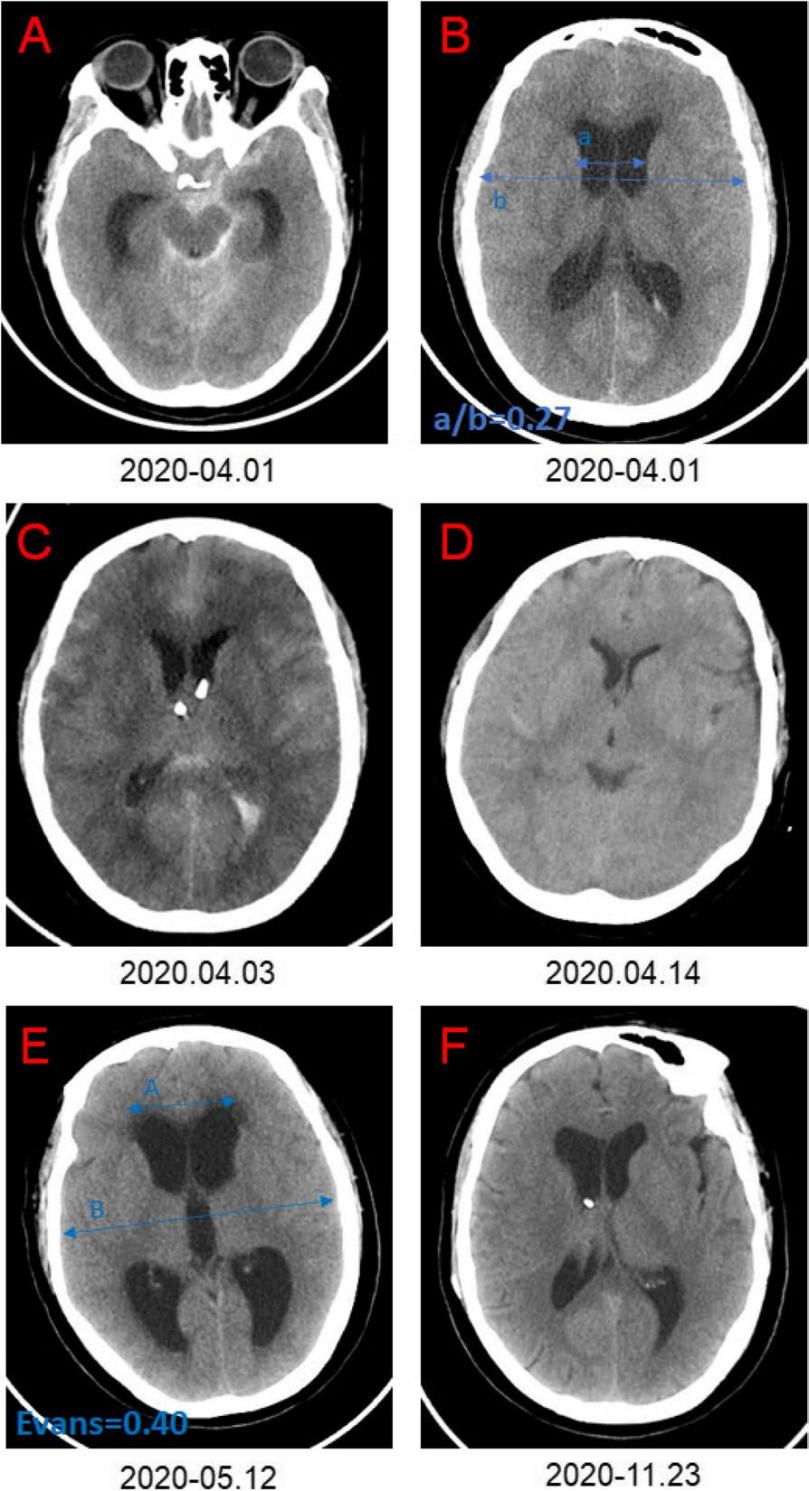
Progression of aSAH-H. A–B indicates subarachnoid hemorrhage complicated with acute hydrocephalus. C Bilateral external ventricular drainage. D Postoperative bilateral external ventricular drainage. E CT indicates chronic hydrocephalus. F Postoperative ventriculoperitoneal shunt
